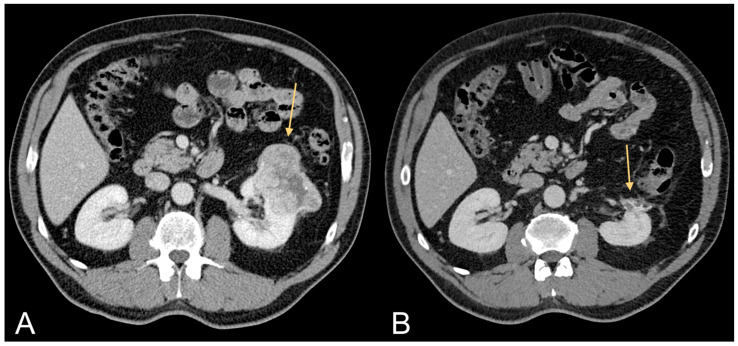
Contrast-enhanced CT images of a 63-year-old male patient with excessive amount of VAT showing ccRCC (light orange arrow in (A)) and follow-up after tumorectomy (light orange arrow in (B)).
